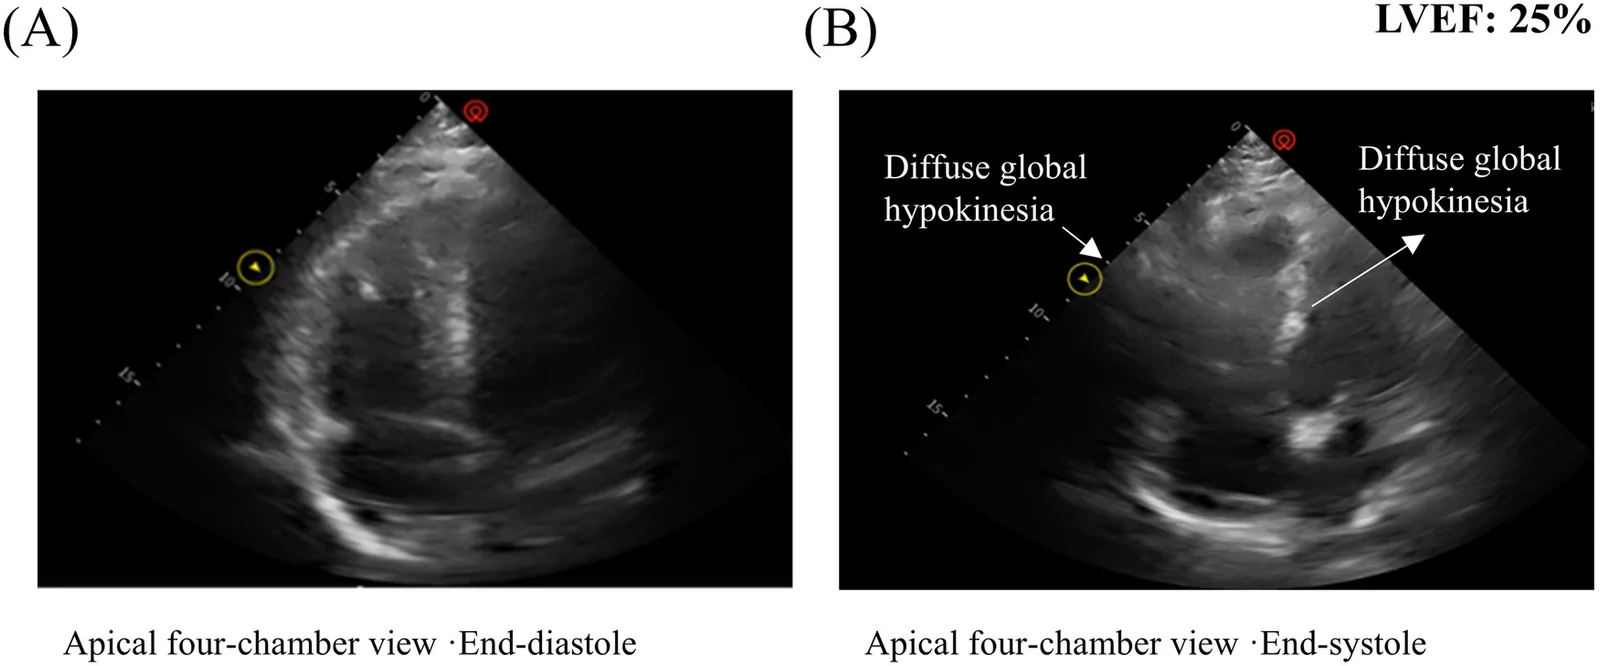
Bedside echocardiography on November 17.
